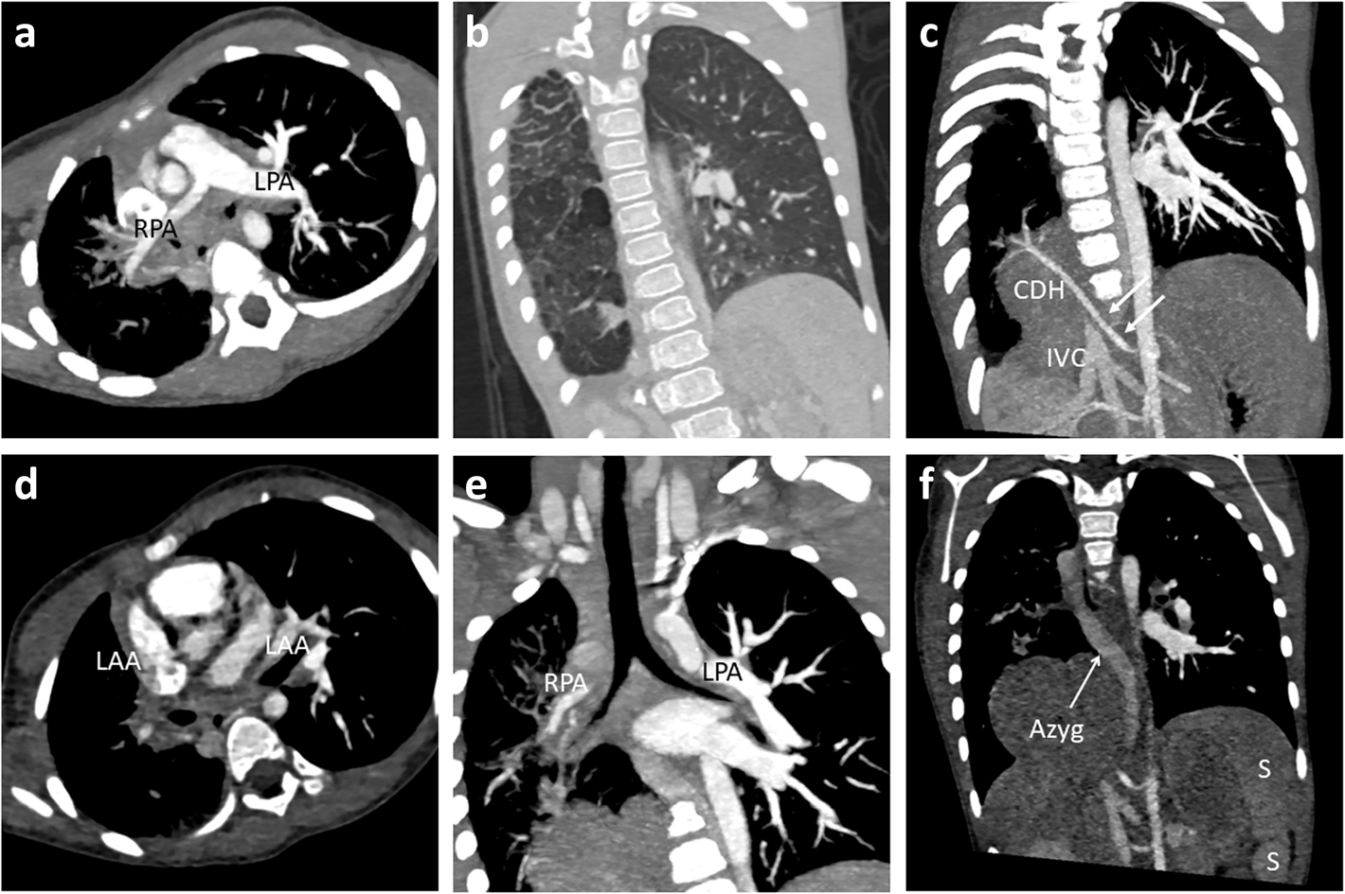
Multiplanar reconstructed cardiac CT images. a Significant size discrepancy between right and left pulmonary arteries. b Right lung parenchymal changes with diffuse interlobular septal thickening and mosaic attenuation. c Systemic-to-pulmonary collateral artery (white arrows) from the coeliac artery supplying the right lung. The hepatic segment of inferior vena cava is interrupted. d Both atrial appendages are long and narrow; characteristic of left atrial isomerism. e Both upper lobar bronchi course below the respective pulmonary arteries (hyparterial bronchi) which is typical of left bronchial isomerism. f Lobulated spleen which is a variant of polysplenia. Also shown is part of the dilated azygous vein. RPA = right pulmonary artery, LPA = left pulmonary artery, CHD = congenital diaphragmatic hernia, IVC = inferior vena cava, LAA = left atrial appendage, Azyg = azygous vein, S = spleen
